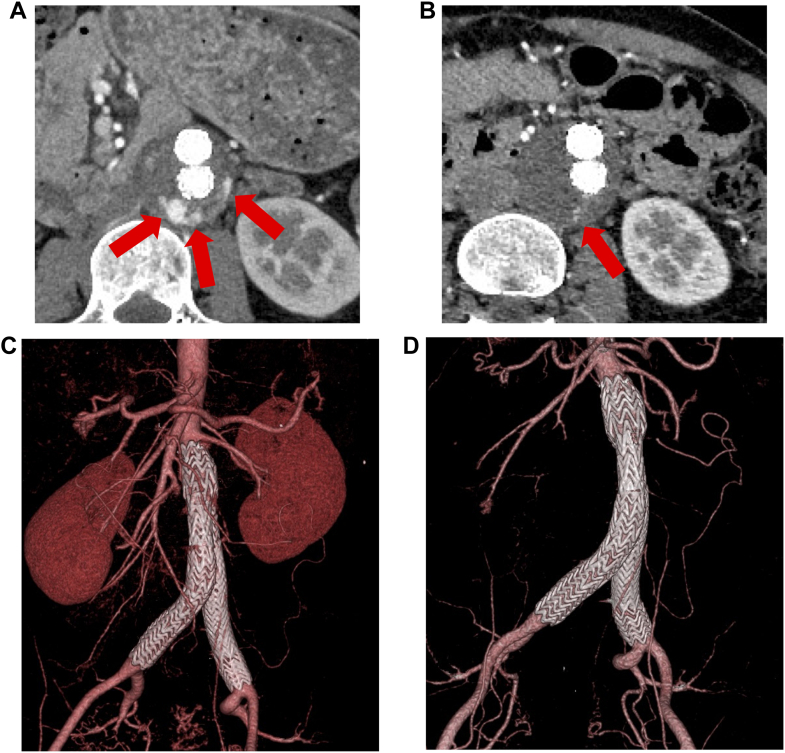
Follow-Up CT After EVAR (A and C) Follow-up computed tomography (CT) at 6 months after endovascular aneurysm repair (EVAR) showed a type II endoleak in the left lumbar artery (red arrow). There was no re-enlargement of the aorta. (B and D) Follow-up CT at 18 months after EVAR showed that the endoleak had decreased in size (red arrow). There was no re-enlargement of the aorta.
